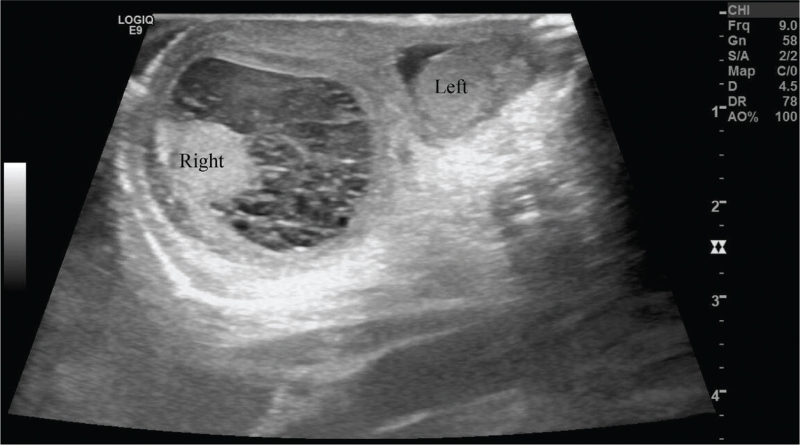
Transverse sonographic image demonstrating the right-sided heterogenous fluid collection with septations suggesting pyocele.
